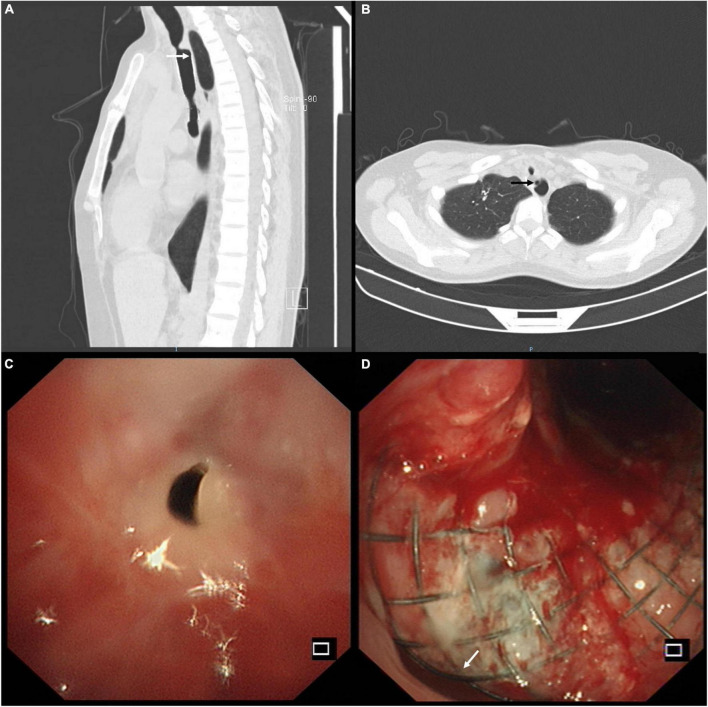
(A) Sagittal chest computed tomography (CT) view showed the severe granulation tissue hyperplasia on the upper edge of the covered self-expandable metallic stent (SEMS) and the shearing force against the anterior esophageal wall (white arrow). (B) Axial chest CT view showed severe granulation tissue hyperplasia and tracheoesophageal fistula (TEF) (black arrow) on the upper edge of the SEMS. (C) The bronchoscopy confirmed severe granulation tissue hyperplasia on the upper edge of the covered SEMS, causing 90% airway obstruction. (D) The bronchoscopy identified the TEF (white arrow) located in the 7 o’clock position after eradicating the severe granulation tissue hyperplasia.
